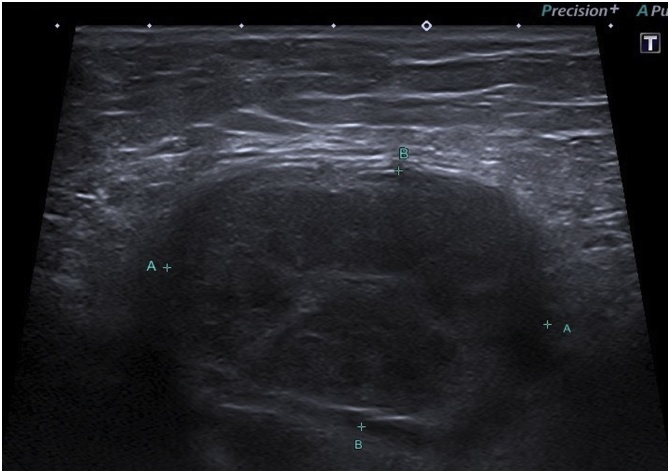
Chronic organized hematoma – ultrasound image eight month after TAPP.
